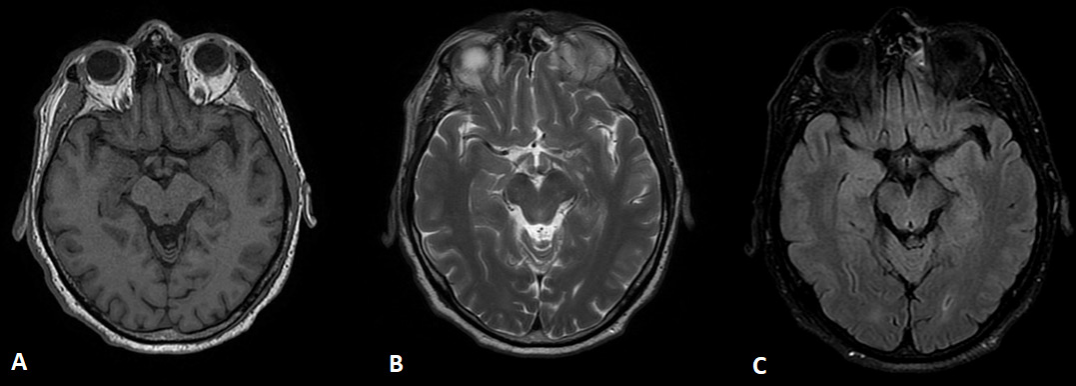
Corresponding axial T1 (panel A), T2 (panel B) and FLAIR (panel C) MR images at the level of the hippocampi. No abnormalities demonstrated.
